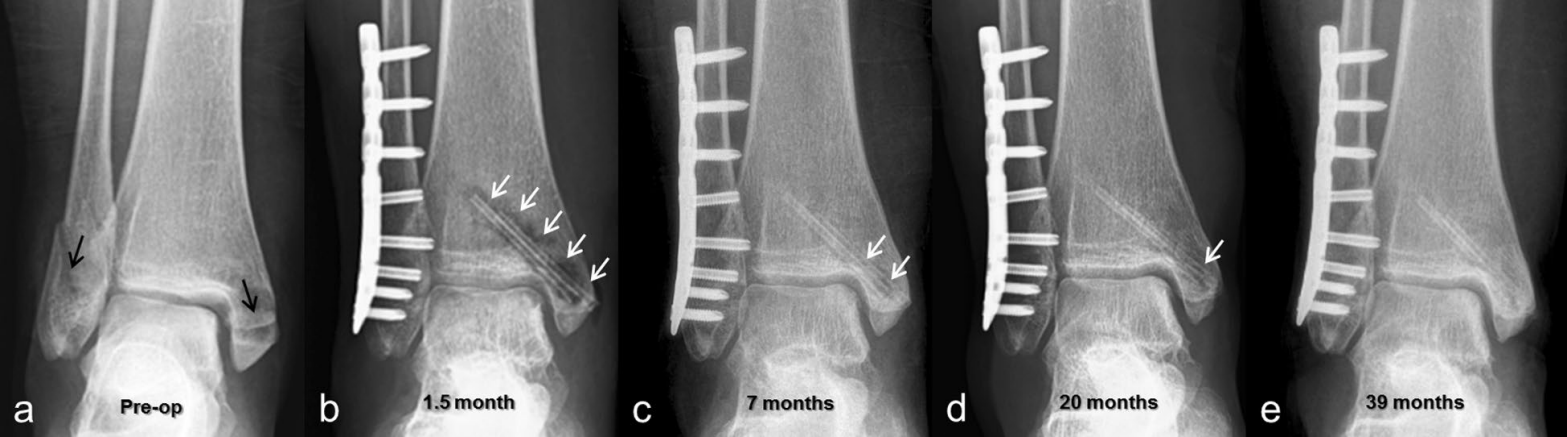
a Preoperative AP ankle radiograph of 45-year-old female patient with a bimalleolar fracture at initial admission (black arrows showing fractures); b Lateral malleolar fracture fixed with plate and screws and medial malleolar fracture fixed with two bioabsorbable Mg screws. At 1.5-month follow-up, gas shadows were observed around screws (white arrows); c At 7th month visit, fracture was united, and significant decrease in gas shadows was seen; d At 20th month follow-up, almost no gas shadows were visible; e At final follow-up, faded silhouette of screws was hardly noticeable
